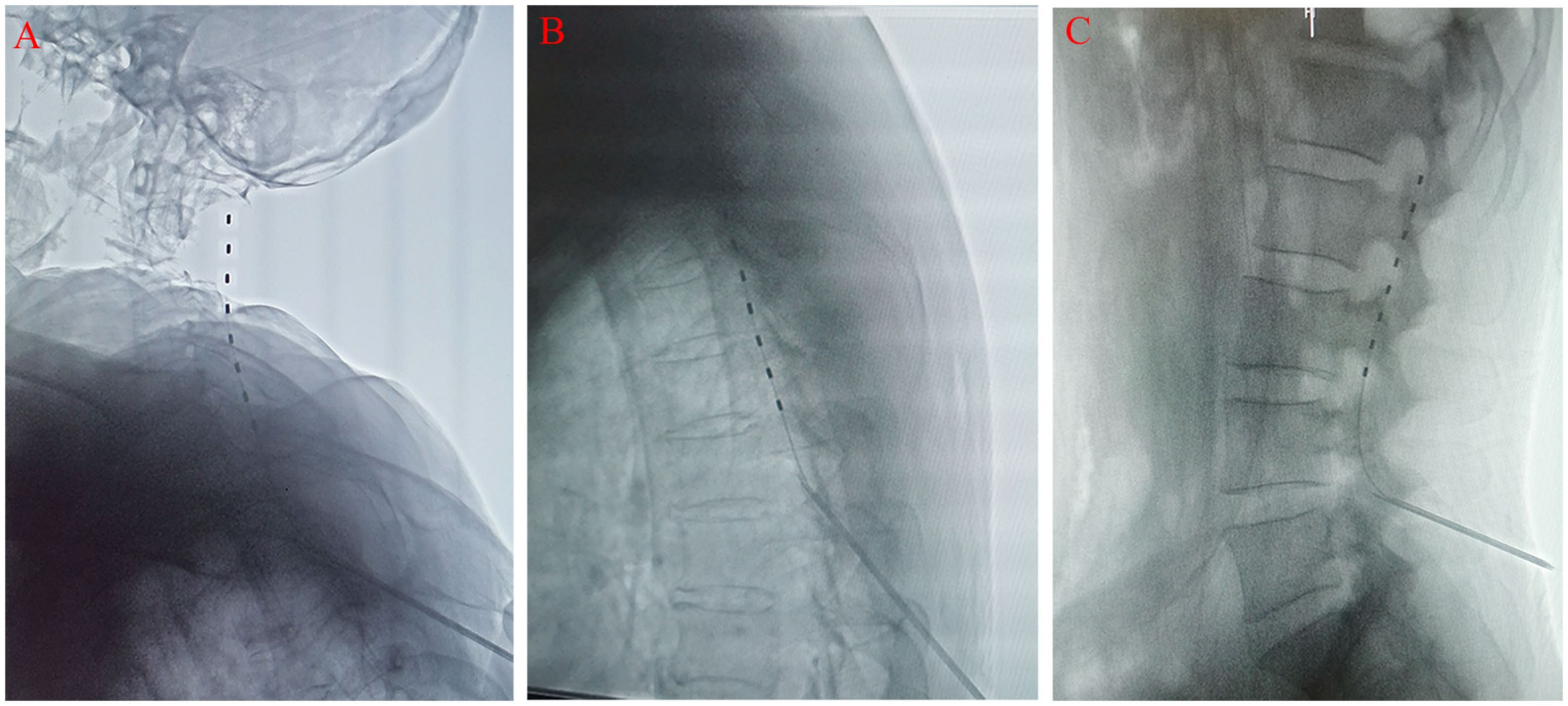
The lateral X-ray film shows that the electrode is located in the posterior epidural space. (A) neck; (B) chest; (C) lumbar.
